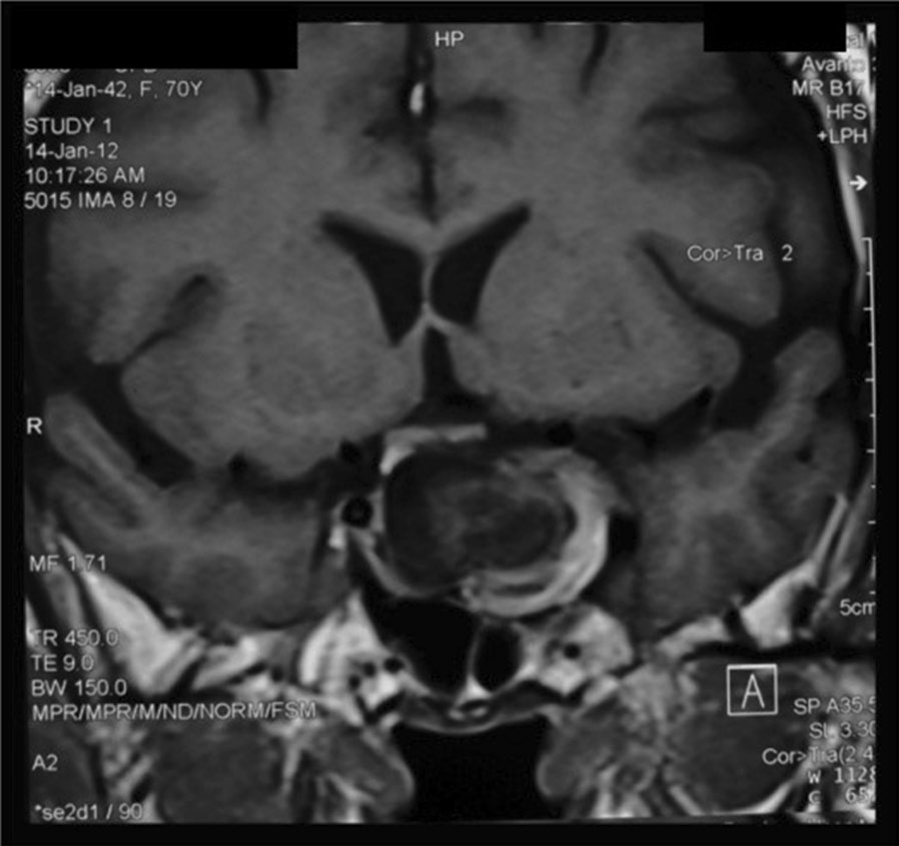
Multi-layered halo like appearance on a coronal section of magnetic resonance imaging
